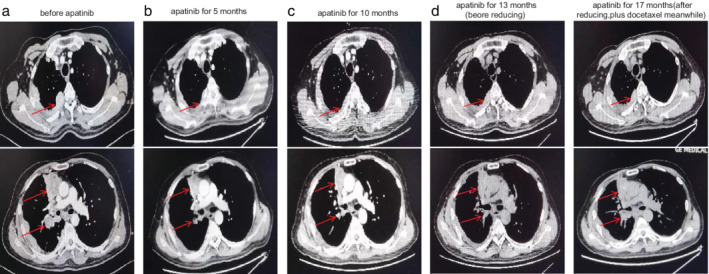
Chest computed tomography (CT) scans before and after apatinib therapy. (a) Before apatinib therapy, a soft tissue mass in the mediastinum and obvious multiple metastases in the lymph nodes of the right hilar and mediastinal, right pleura, and right lung were visible. (b) After five months of apatinib, chest CT showed a remarkable reduction of the mediastinal mass and metastatic lesions. (c) After 10 months of apatinib treatment, the mediastinal mass and metastatic lesions remained stable. (d) After 13 months of treatment with apatinib, the mediastinal lesion had progressed, but still remained stable overall. (e) After 17 months of apatinib, a chest CT showed progressive disease (PD). The patient had received treatment with apatinib for a total of 17 months.
